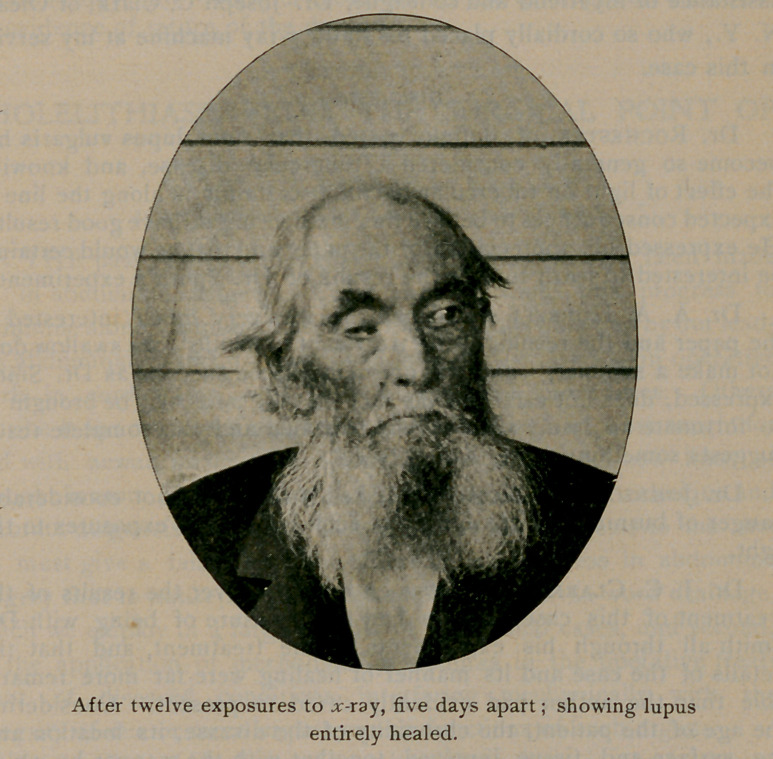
# Lupus Vulgaris of Fifteen Years’ Standing Successfully Treated and Cured by Exposure to X-Ray1Read before the Cattaraugus County Medical Society, Salamanca, N.Y., November 20, 1900.
